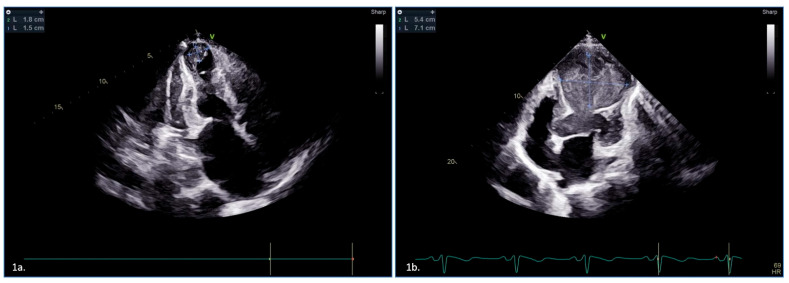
Echocardiographic demonstration of thrombus in two cases managed by different anticoagulation regimens. (1a)—two-dimensional echocardiography in apical view showing homogenous echo dense mass (1.8 × 1.5 cm) at apex of left ventricle, suggestive of thrombus. (1b)—two-dimensional echocardiography in apical view showing large echo dense mass (5.4 × 7.1 cm) at apex of a dilated and akinetic left ventricle, suggestive of thrombus.
